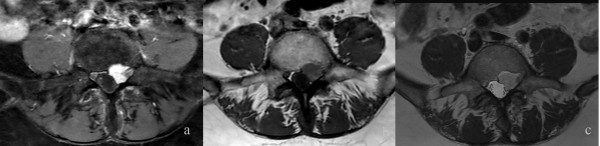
Magnetic resonance imaging findings. The T2-TSE (b) and the T1-TSE (c) sequence reveals an intraspinal, extradural soft-tissue mass in the left L4 - S1 paravertebral region. It is compressing the nerve roots of L4 and L5. The T1 SPIR (a) sequence with contrast medium shows the homogenous enhancement of this lesion.
